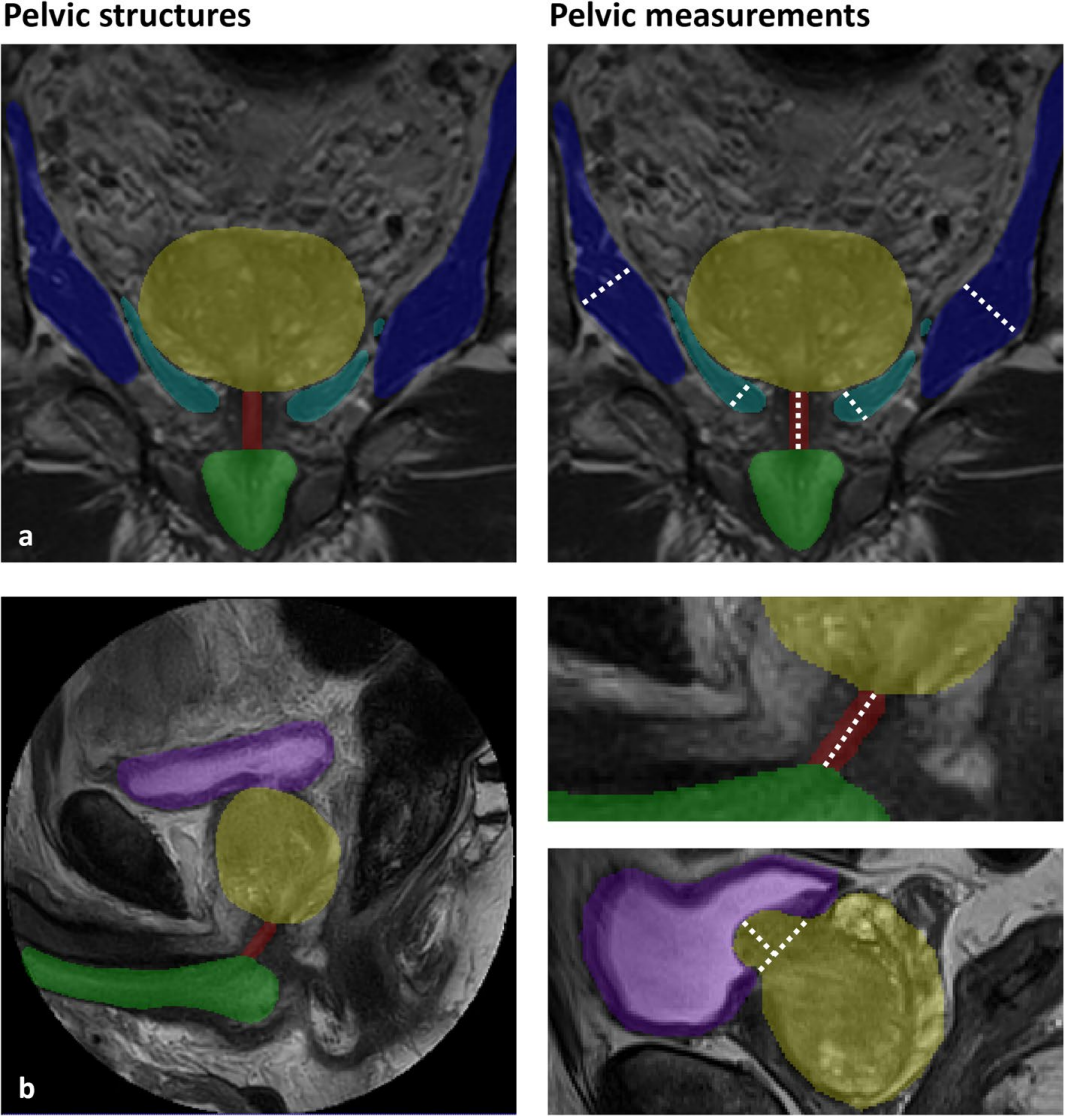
Pelvic structures and pelvic measurements in coronal direction (a) and sagittal direction (b) on T2-weighted images. Pelvic structures include the bladder (purple), corpus spongiosum (green), prostate (yellow), membranous urethra (red), obturator internus muscle (dark blue), and levator ani muscle (cyan)
